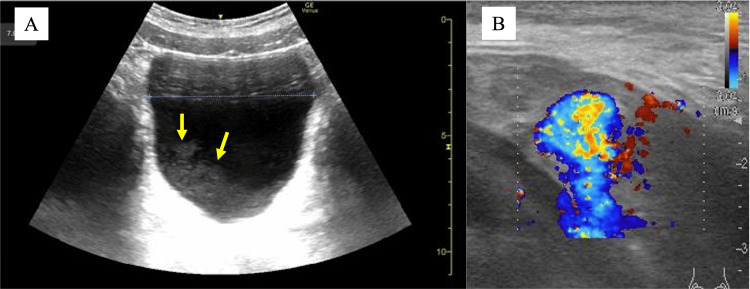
Abdominal ultrasound findings. (A) Abdominal ultrasound demonstrating intravesical blood clots (the yellow arrow indicates the blood clot). (B) Doppler ultrasonography demonstrating arterial flow within an intraluminal mass in the bulbar urethra.
